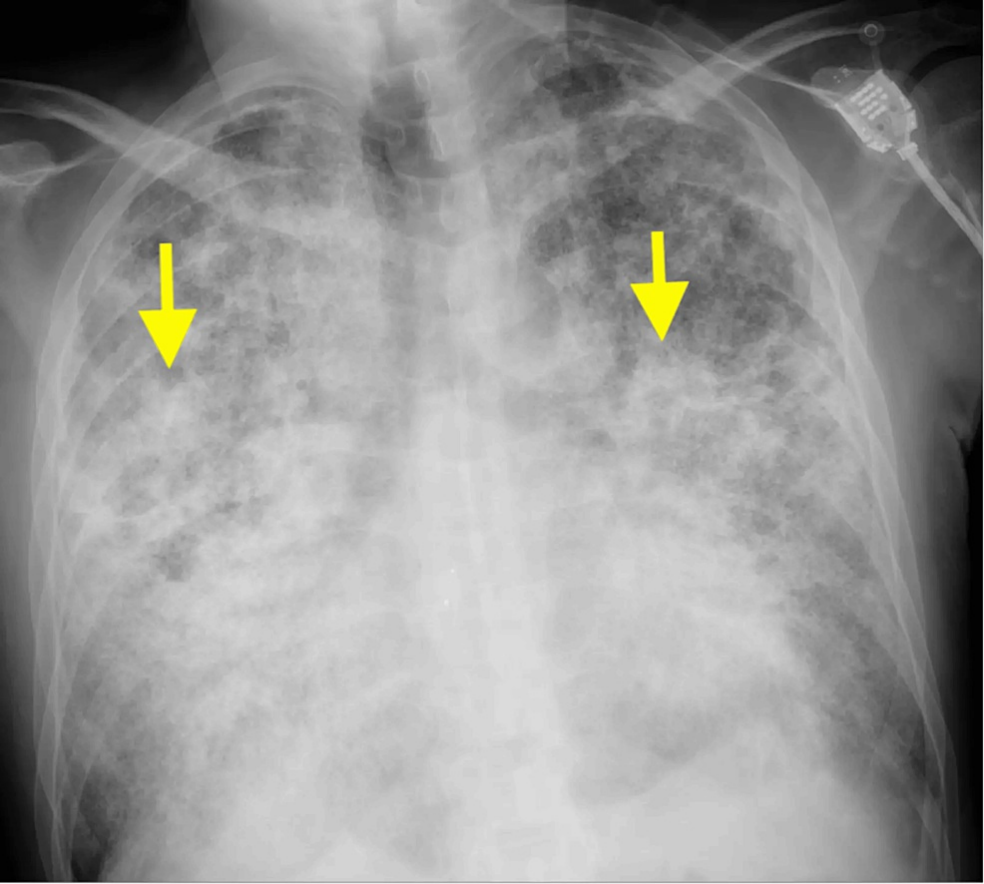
Chest radiograph showing fine sand-like infiltrates (yellow arrows) in both lungs
